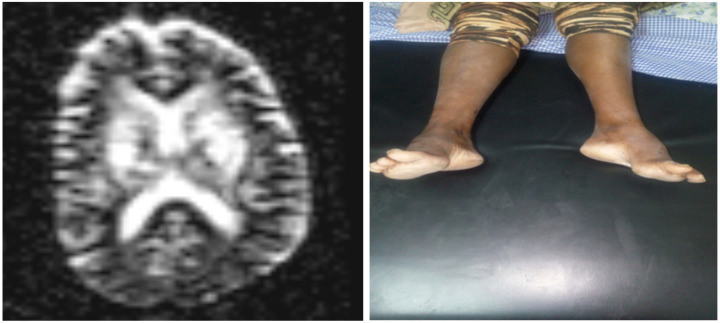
a) Diffusion-weighted image (DWI) of apparent diffusion coefficient (ADC) map shows no restricted diffusion in the affected areas of the brain; b) Bilateral pes-cavus, likely due to chronic lower limbs weakness
